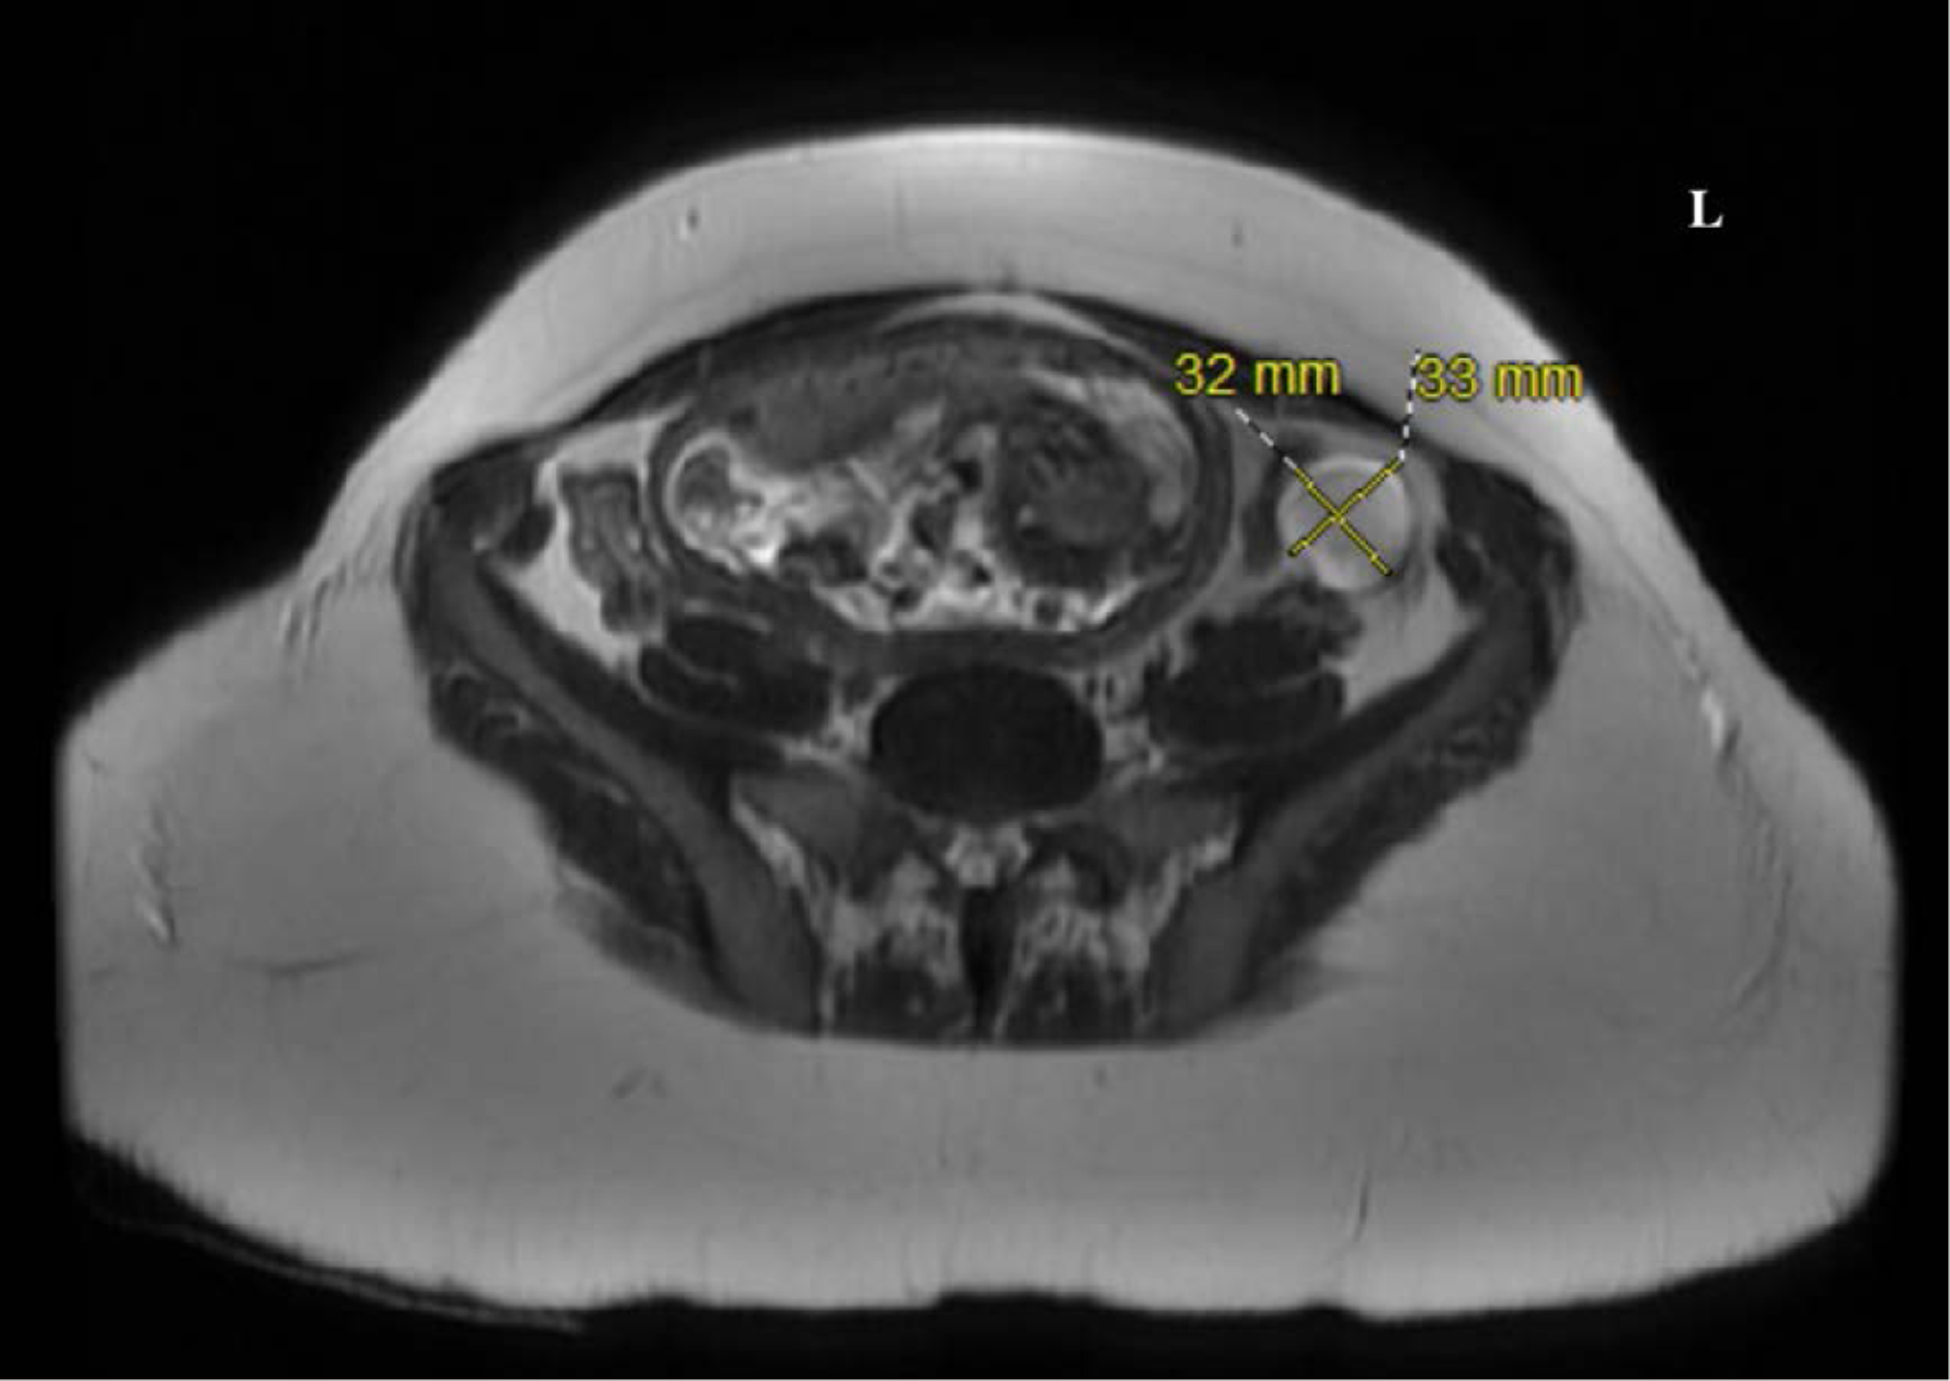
Pelvic MRI demonstrating intrauterine gestation and a left ovarian complex mass with layering debris approximately 33mm × 32mm in size
